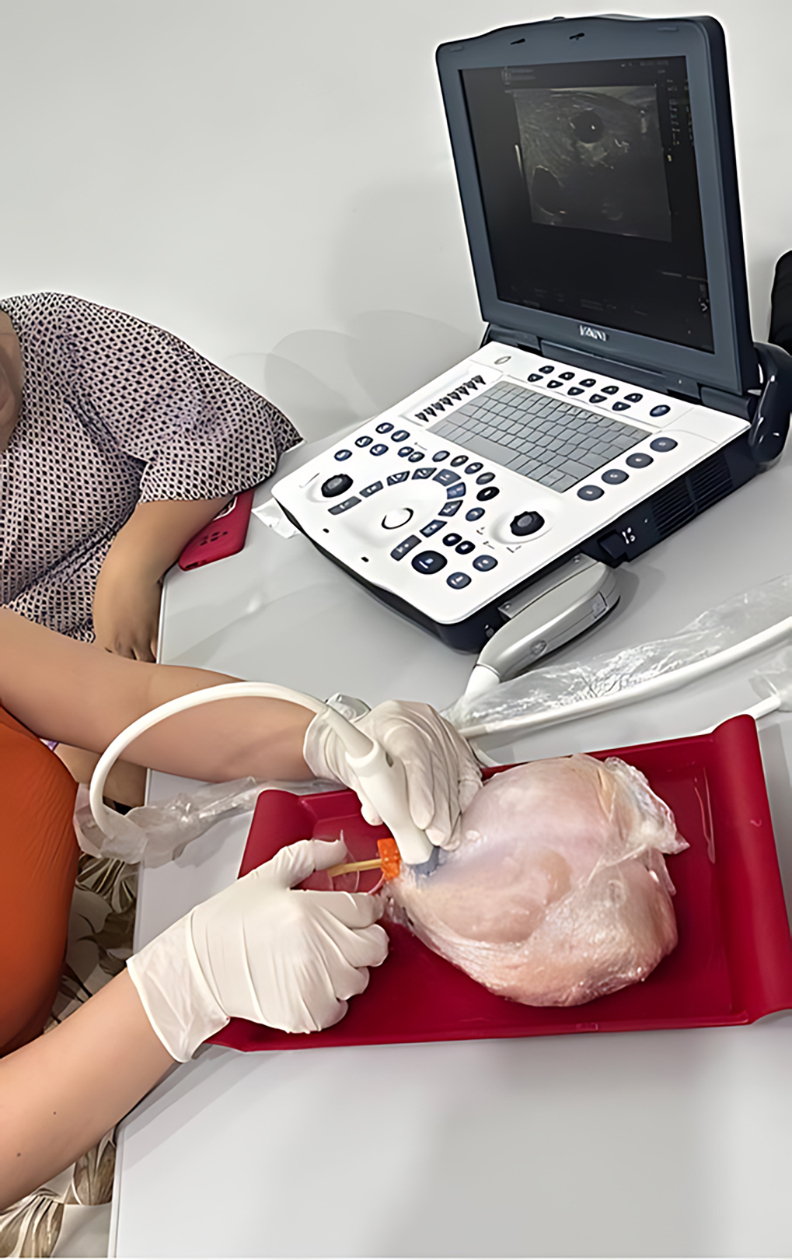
Training nursing professionals from the Department of Renal Replacement Therapy, Fundação Hospital de Clínicas Gaspar Vianna, in ultrasound-guided puncture. Source: authors’ files.
